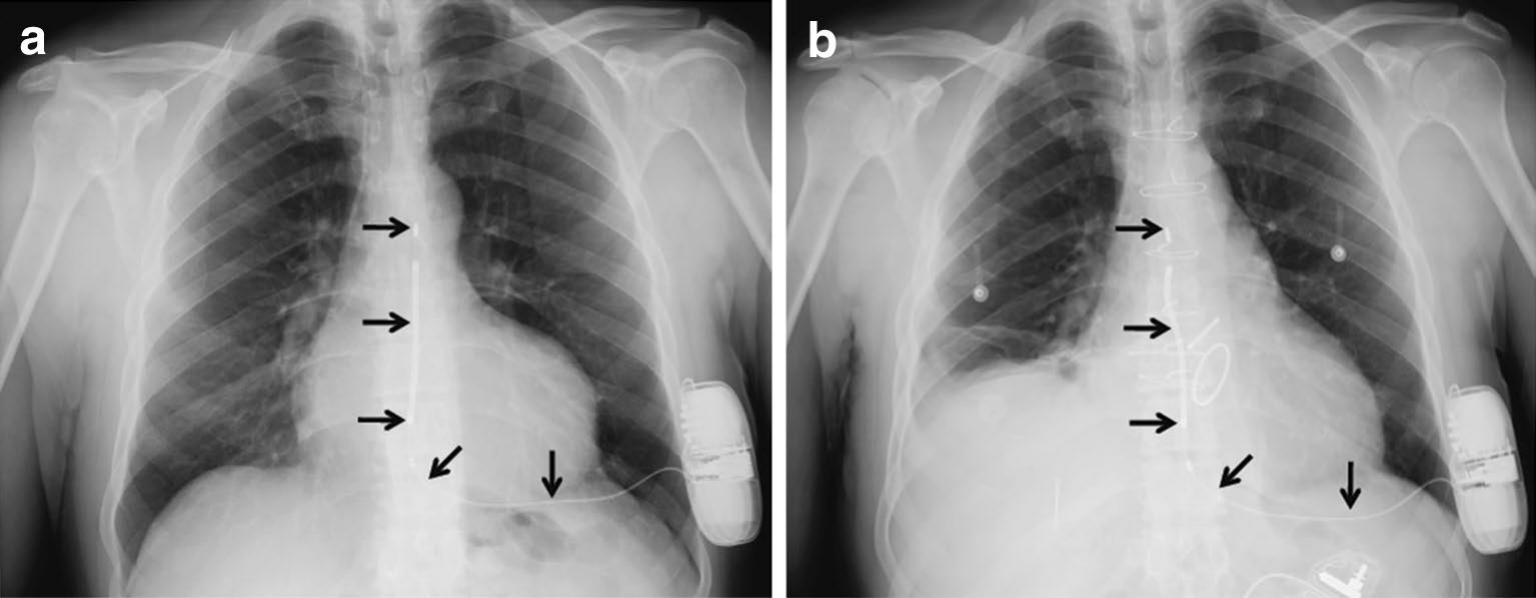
Comparison of chest X-rays before (a) and after (b) the surgical procedure confirmed an adequate positioning of the lead
